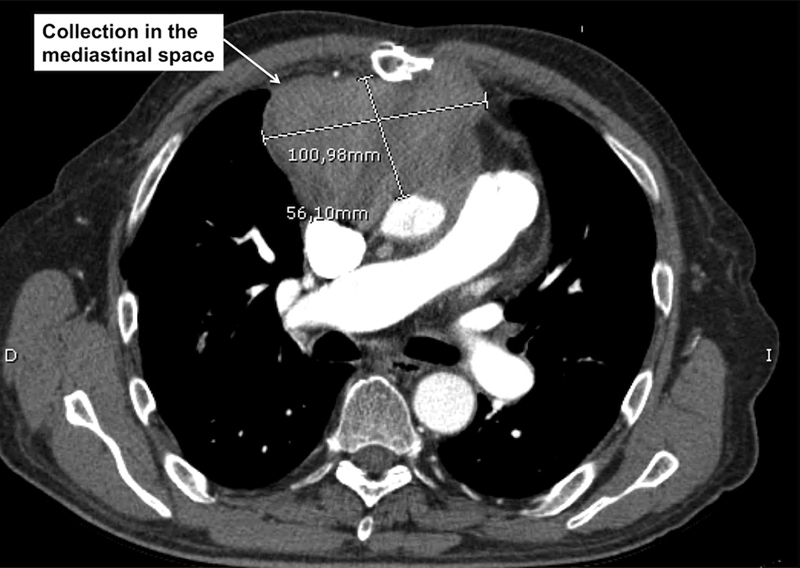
Computed tomography scan with intravenous contrast. Axial section showing a big collection in the mediastinal space.
